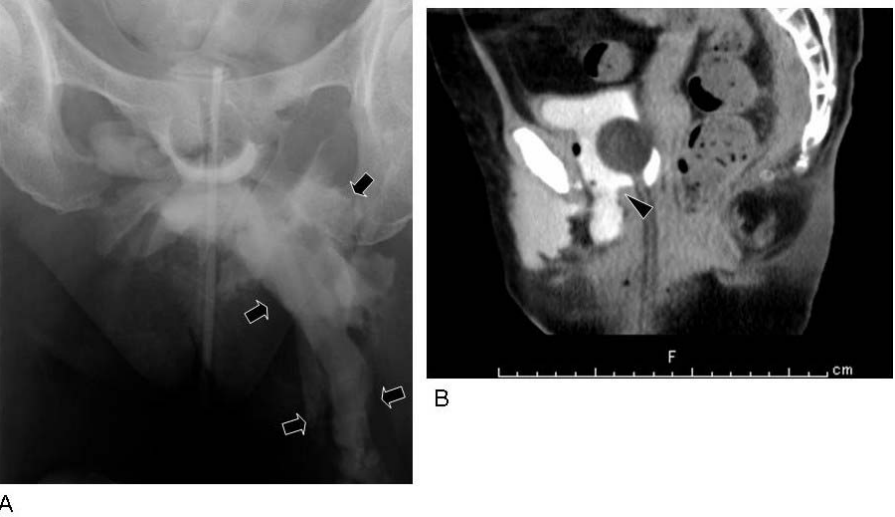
Retrograde cystography (A) performed on day 16 and sagittal reconstruction image of CT cystography (B) performed later. A: The urinoma extending to the left thigh is enlarged when compared with day 4 (arrows). B: In the sagittal reconstruction CT image, the laceration is more clearly defined (arrowhead). The base of the bladder is positioned at the pubic bone inferior margin lower than the image obtained on day 4.
